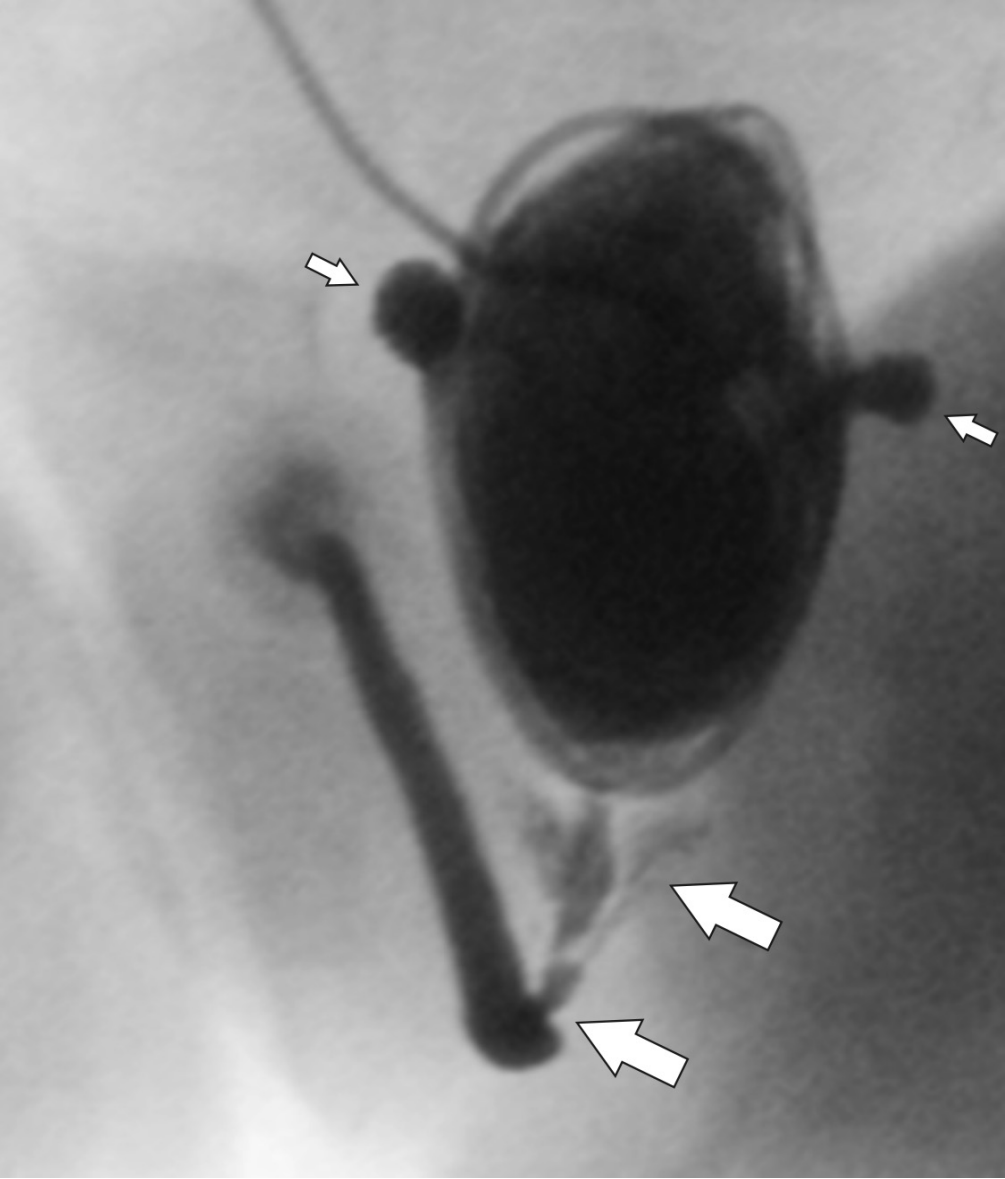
The voiding cystourethrosonography shows a urethral obstruction with a pre-stenotic dilatation of the urethra and the seminal vesicles (thick arrows). The patient also had a thickened bladder and two bladder diverticuli (small arrows).
